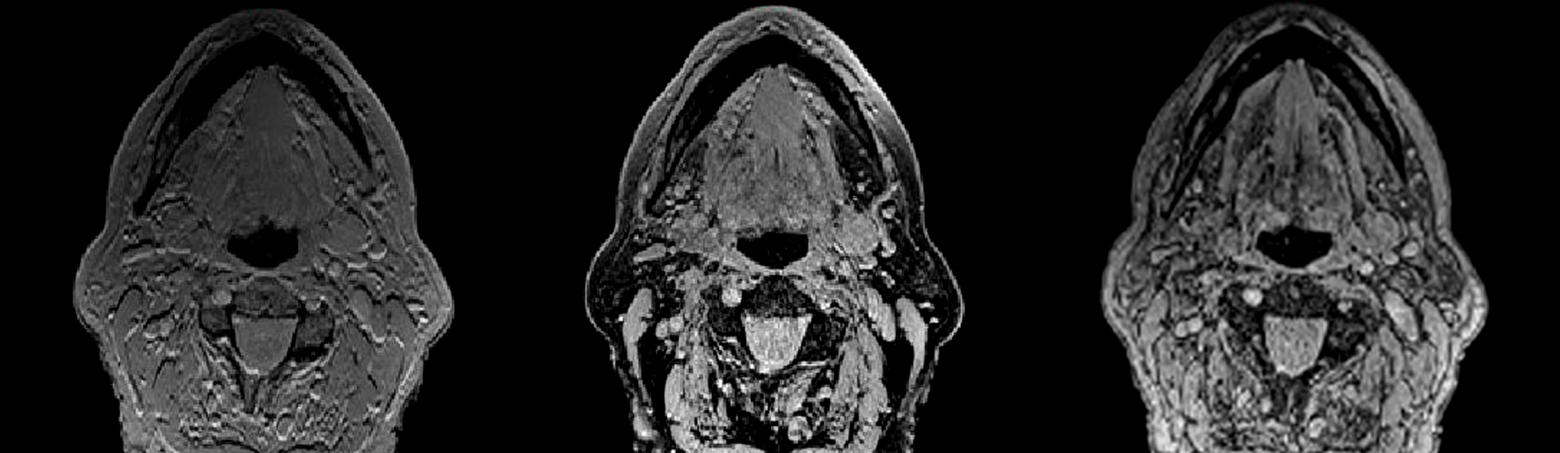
Axial views of the three different Black Bone sequences. From left to right: standard Black Bone MRI sequence, Black Bone with quick FATSAT + GRAPPA, and Black Bone out of phase + quick FATSAT. More noise is visible in the middle and right images and these images also show more soft tissue contrast.
